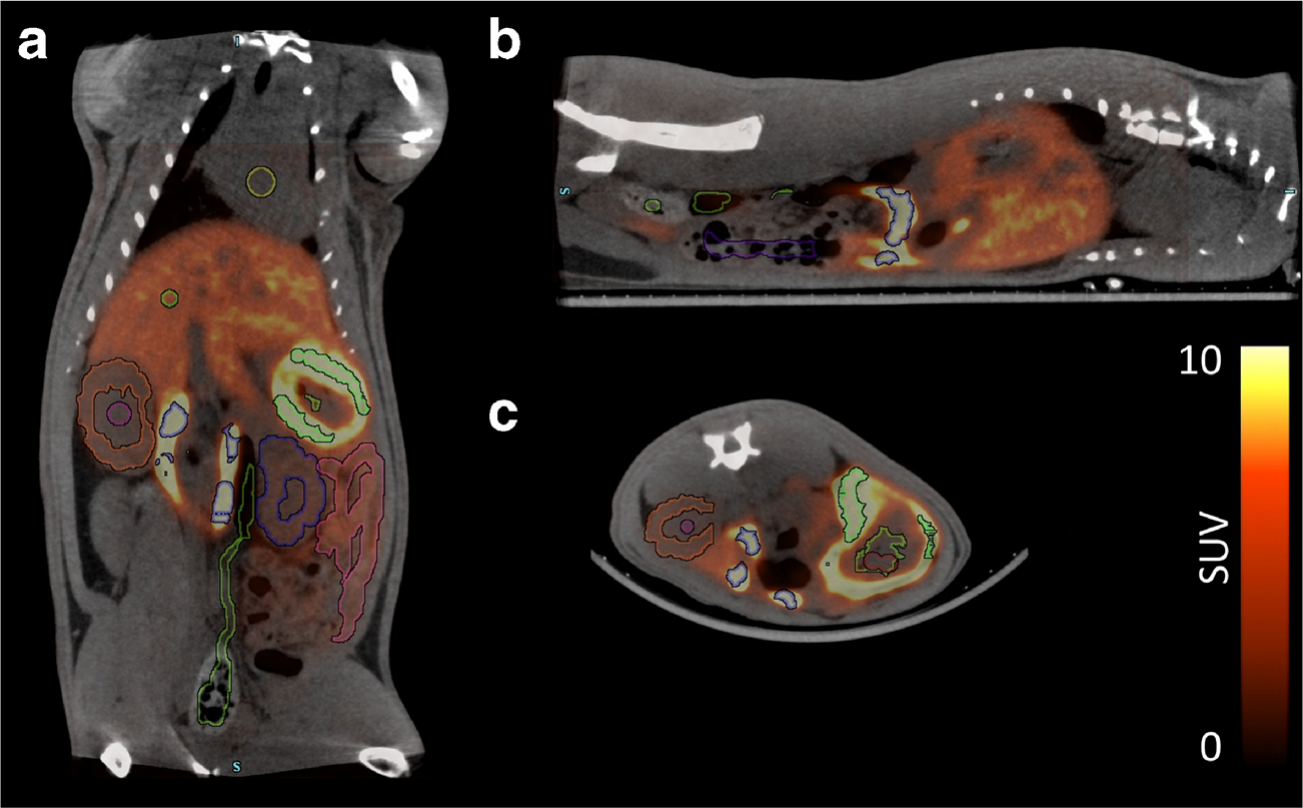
a Coronal, b saggital and c transversal view of a representative PET/CT scan with the manually defined volumes of interest.
